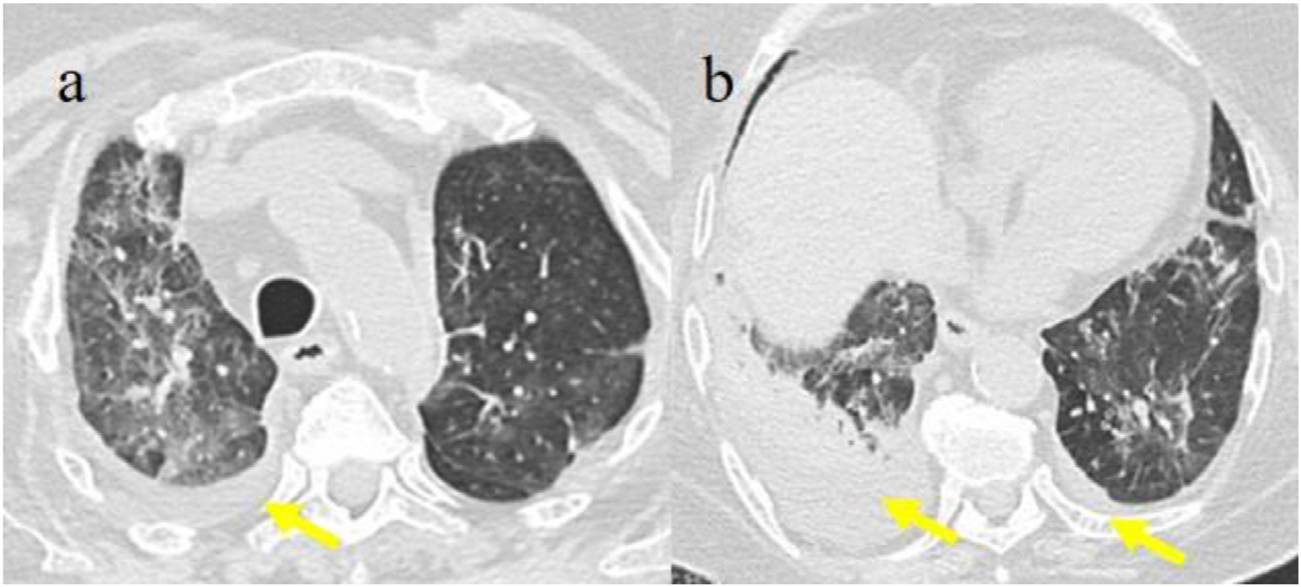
Chest CT performed after other 10 days that showed a mild reduction of the consolidations areas with increased appearance of the right pleural effusion (yellow arrow in the image a and b) and a small pleural effusion also on the left side (yellow arrow in the image b).
